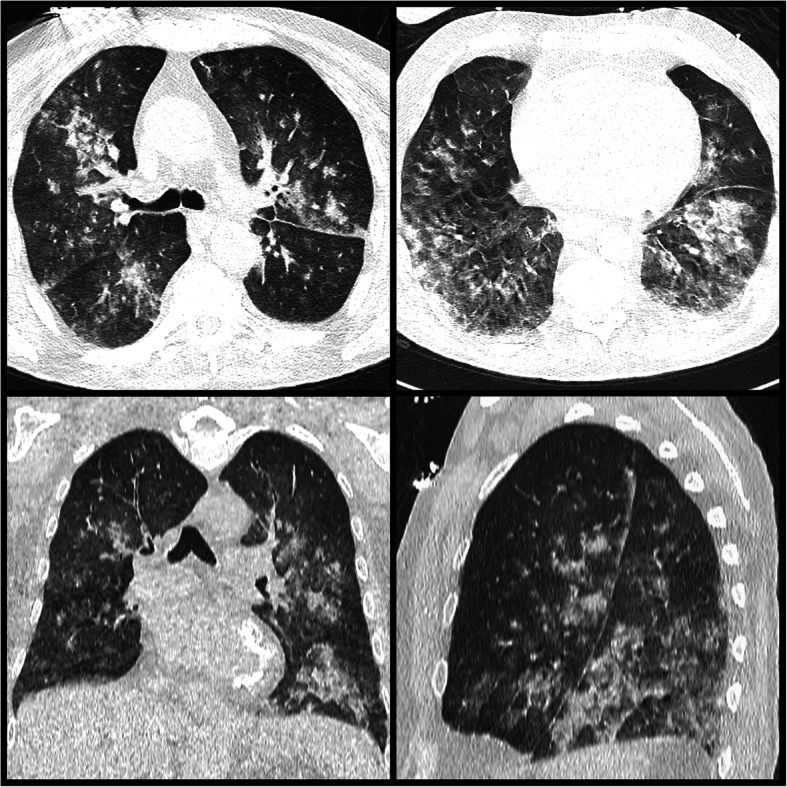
Chest CT-scan image of the patient on day 1. Axial, coronal, and sagittal plane CT images of the lung, with a 3-mm slice width made by Siemens Somatom Definition Device. Alveolar atelectasis from base to apex
